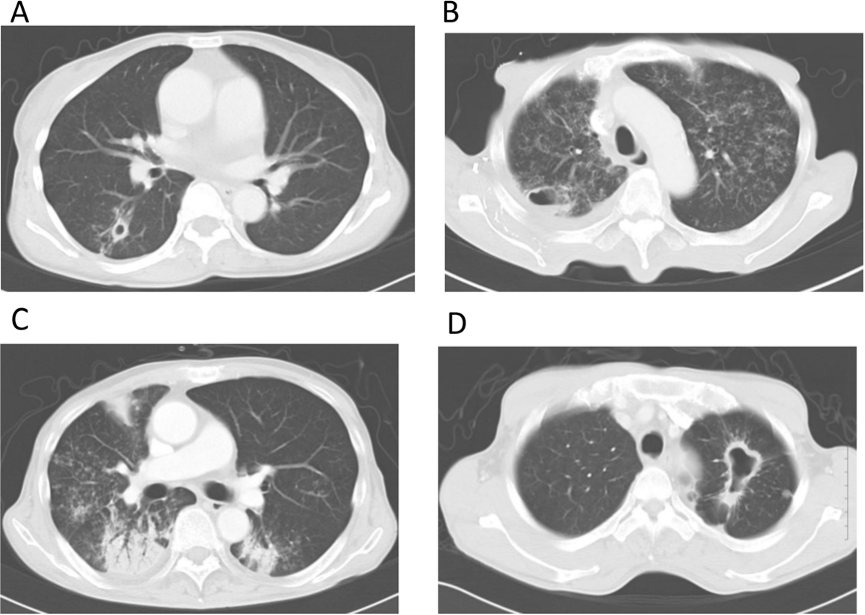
Thoracic computed tomography findings in patients with MTB or MK infection. A: Cavity formation with thick wall. B: Infiltration shadow in bilateral lungs and cavity formation in the right upper lobe. C: Infiltration shadow with patty follicular spot in the bilateral lungs. D: Cavity formation with thick wall in the left upper lobe.
